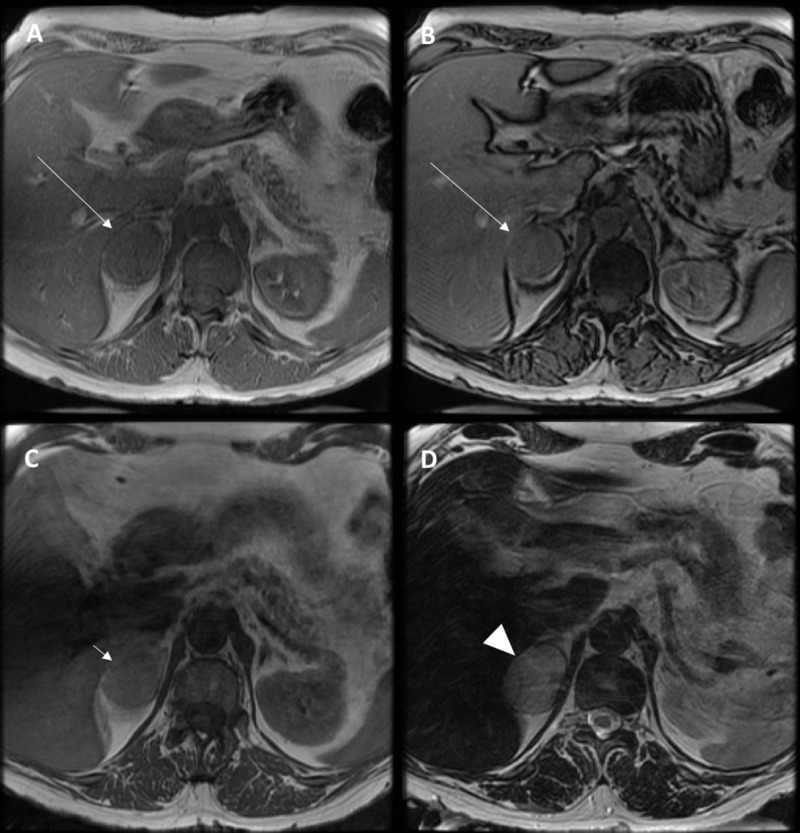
Axial MRI of the upper abdomen including (A) in-phase and (B) out-of-phase (FSPGR), (C) GRE T1, and FSE T2 (D) Note that the right adrenal mass does not decrease in signal on out-of-phase imaging (long white arrow), has an intermediate T1 signal (short white arrow) and a high T2 signal (white arrowhead). MRI: magnetic resonance imaging, FSPGR: fast spoiled gradient echo, GRE: gradient echo, FSE: fast spin echo
